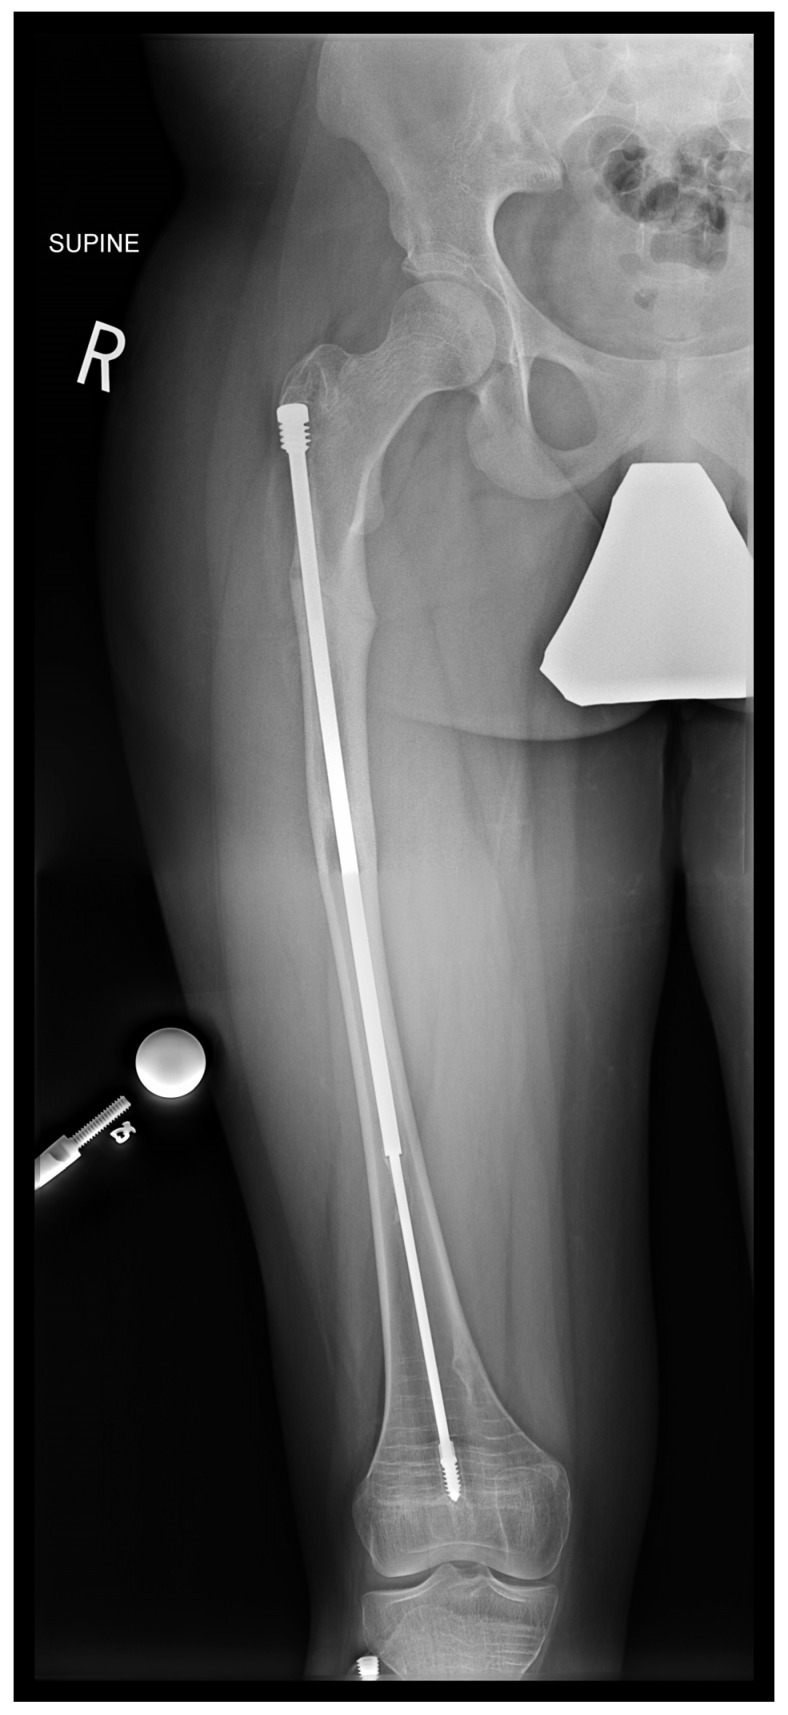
Pre-operative X-ray showing right femur stress fracture.
